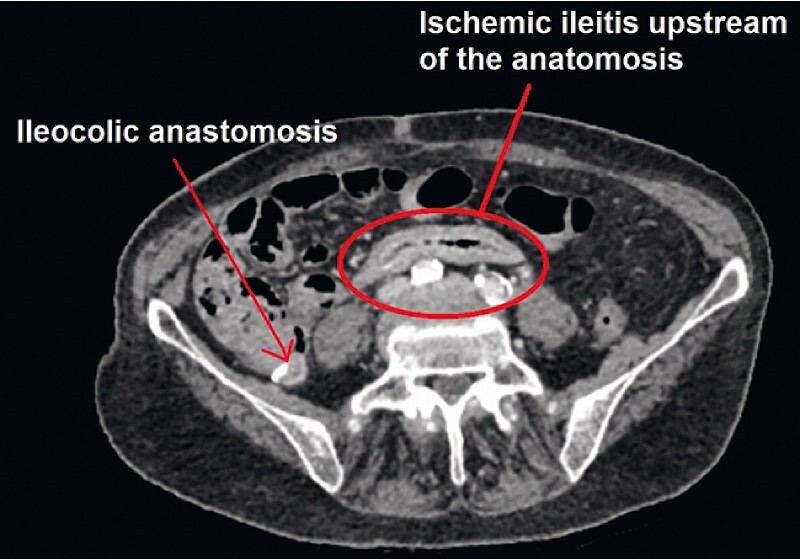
Abdominopelvic computed tomography scan showing thickened aspect of the ileal mucosa in front of the ileocolic anastomosis, corresponding to postsurgical ischemic damage of the ileum.
